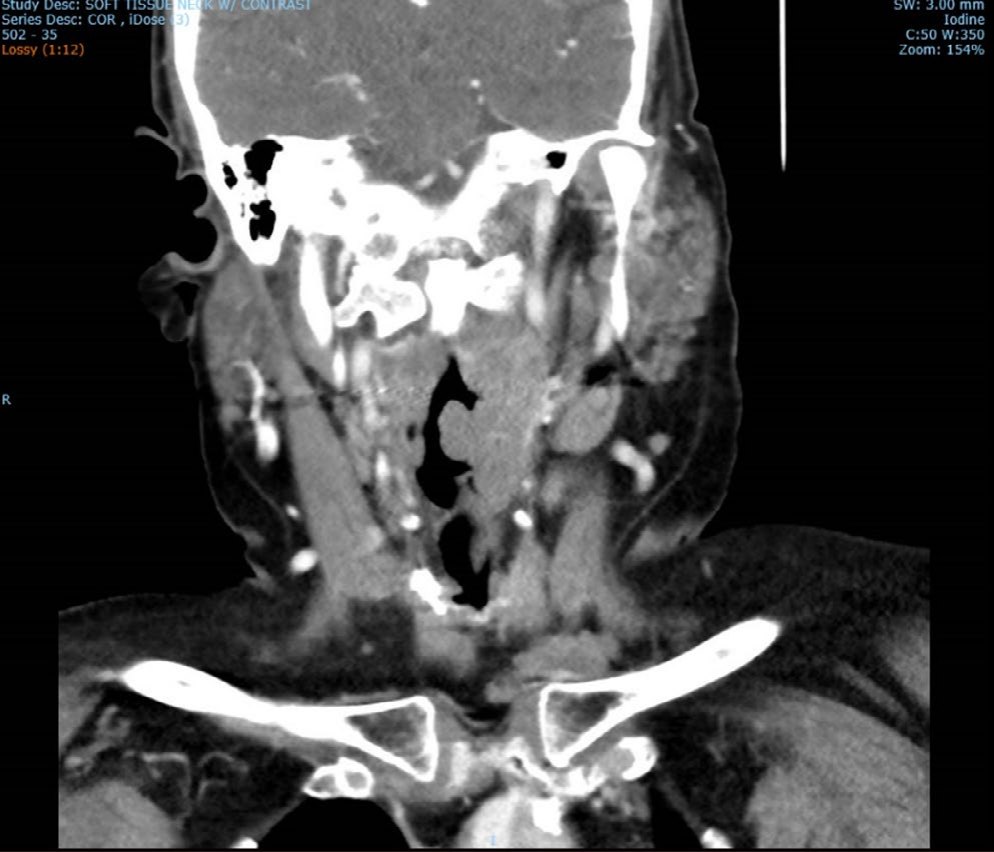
CT soft tissue coronal of laryngeal lesion
